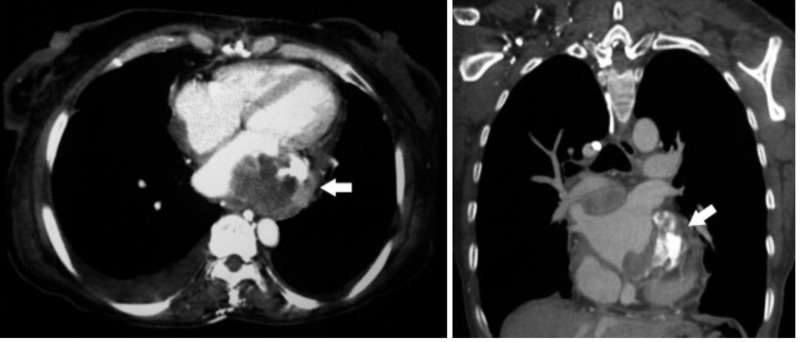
Thoracic computed tomography (CT) showing an irregular left atrial mass (arrow) attached to the posterior wall
